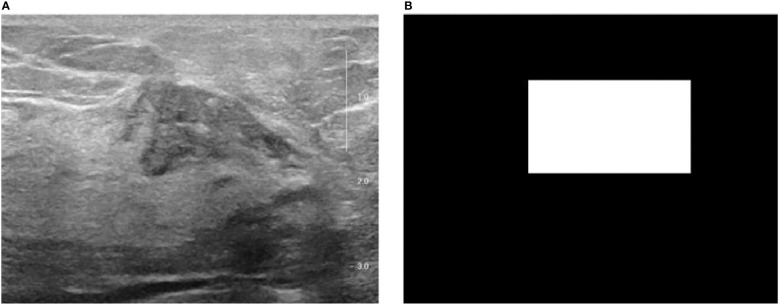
The original breast ultrasound image and their corresponding masked image. (A) Original image; (B) Masked image.
